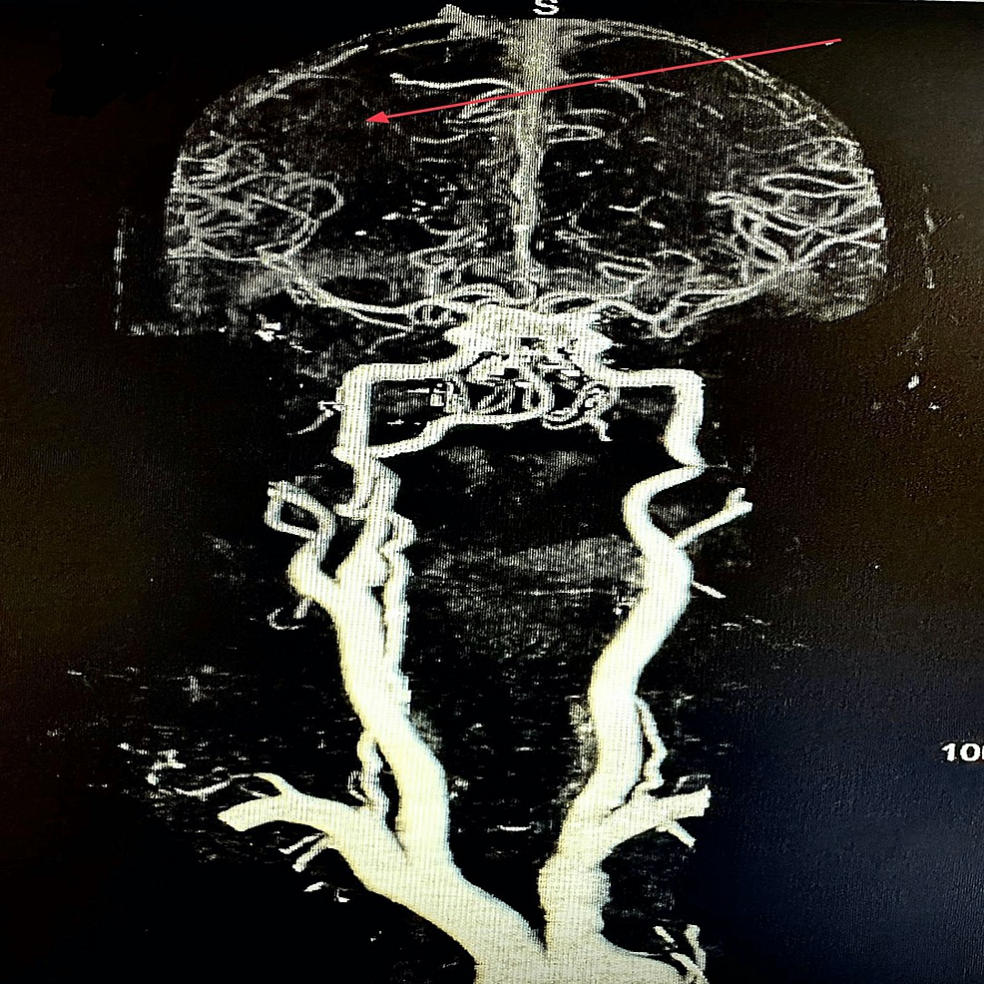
CT angiogram of the head and neck with and without contrast showing decreased right MCA circulation compared to left MCA. CT, computed tomography; MCA, middle cerebral artery
